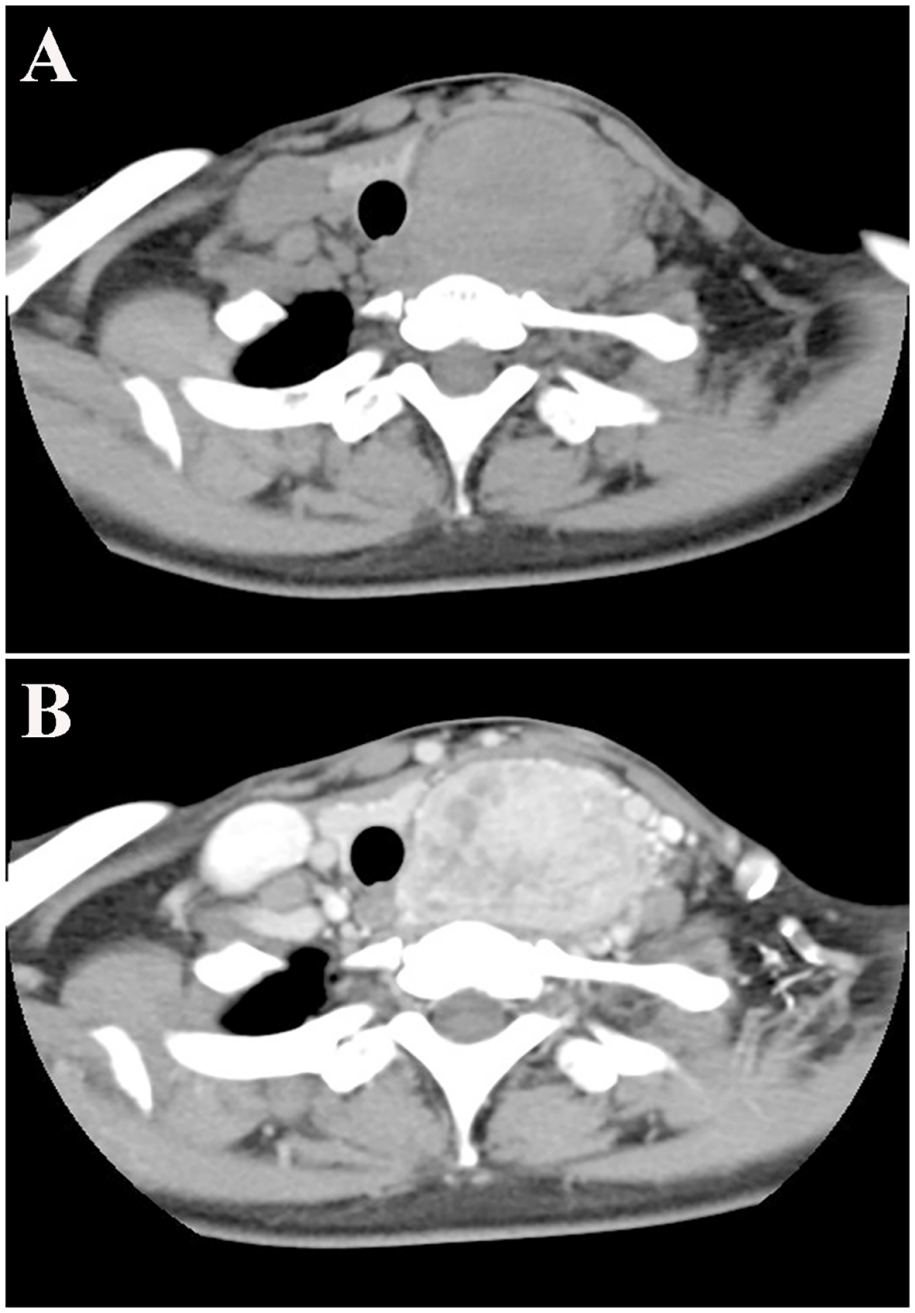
Cervical computed tomography scan (A, B).
